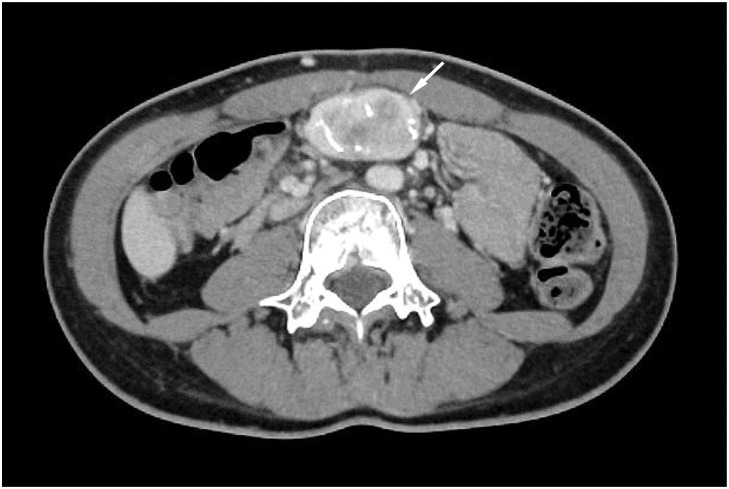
Contrast-enhanced CT of the abdominal wall mass. Axial contrast-enhanced CT demonstrates a 45-mm well-circumscribed solid mass with coarse internal calcifications located between the supraumbilical and umbilical abdominal wall (arrow). The lesion abuts the posterior aspect of the abdominal wall without any evidence of intraperitoneal extension.
